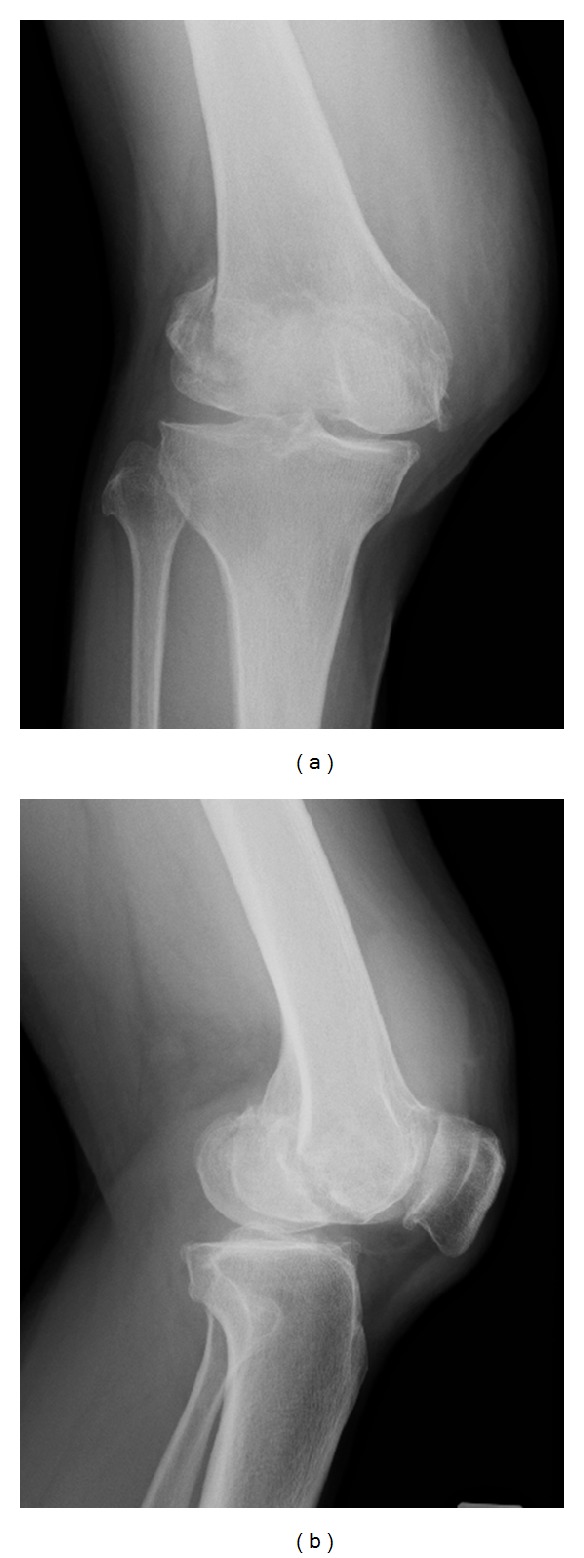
Initial posttraumatic anteroposterior (a) and lateral (b) radiographs of the right knee.
